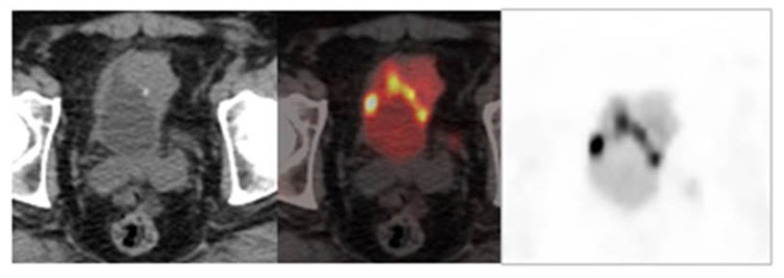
Image obtained with FDG-PET/CT after the administration of a diuretic.
